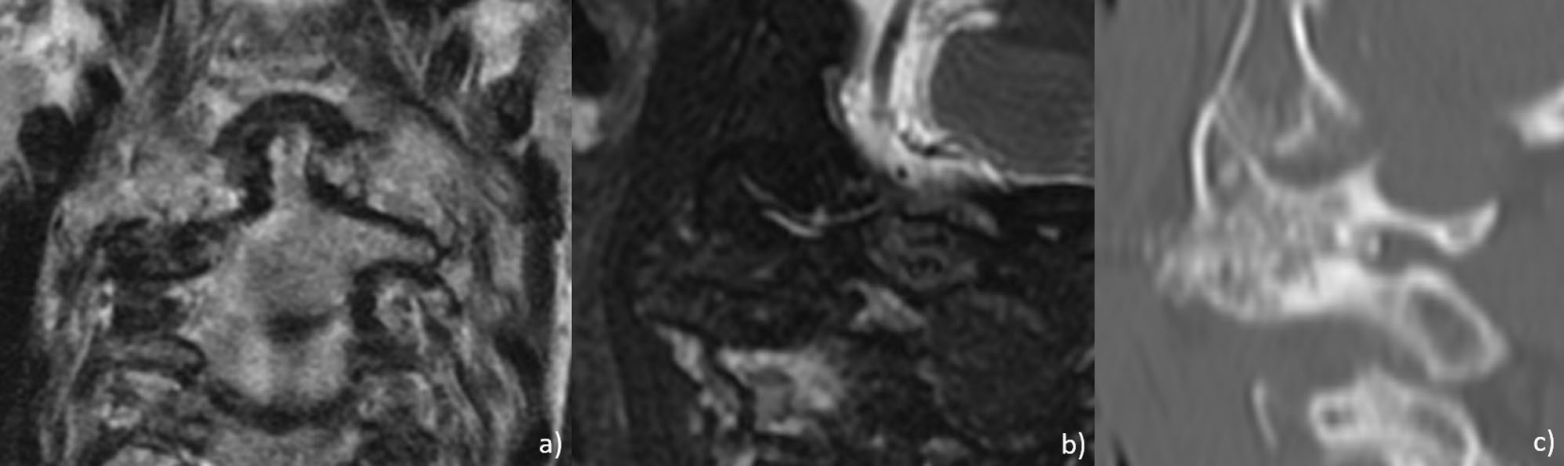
Coronal T2 (a), sagittal fat-saturated T2 (b) and sagittal CT (c) MRI images showing erosion and partial fusion of left lateral atlantoaxial joint.
